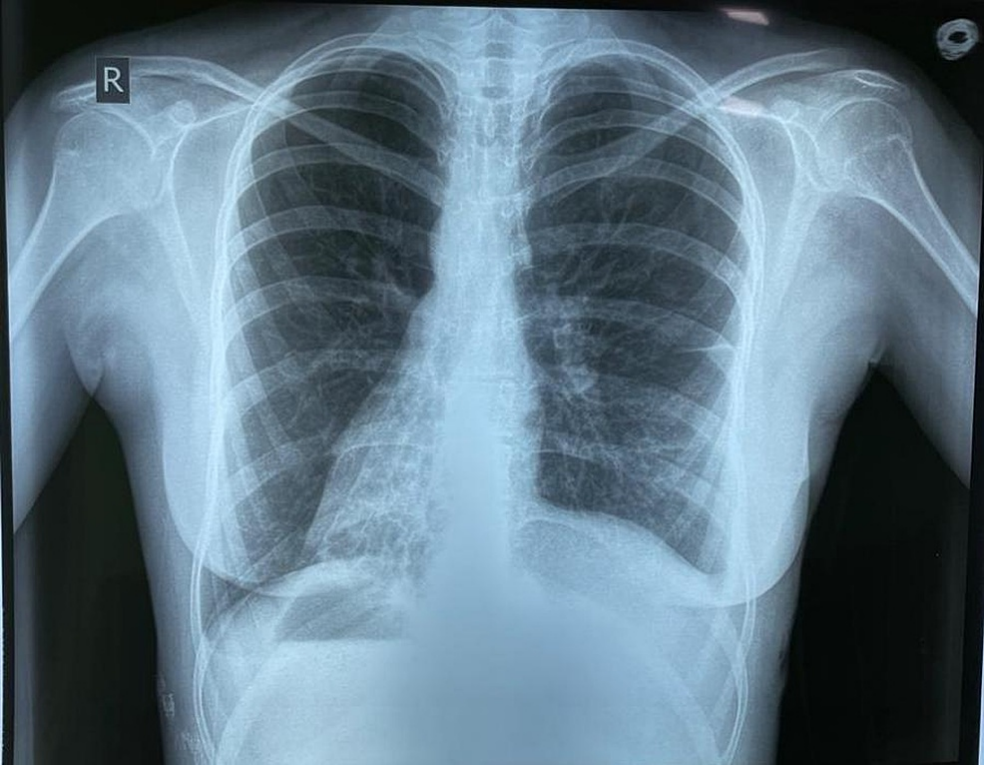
Chest X-ray taken three weeks postoperatively.
